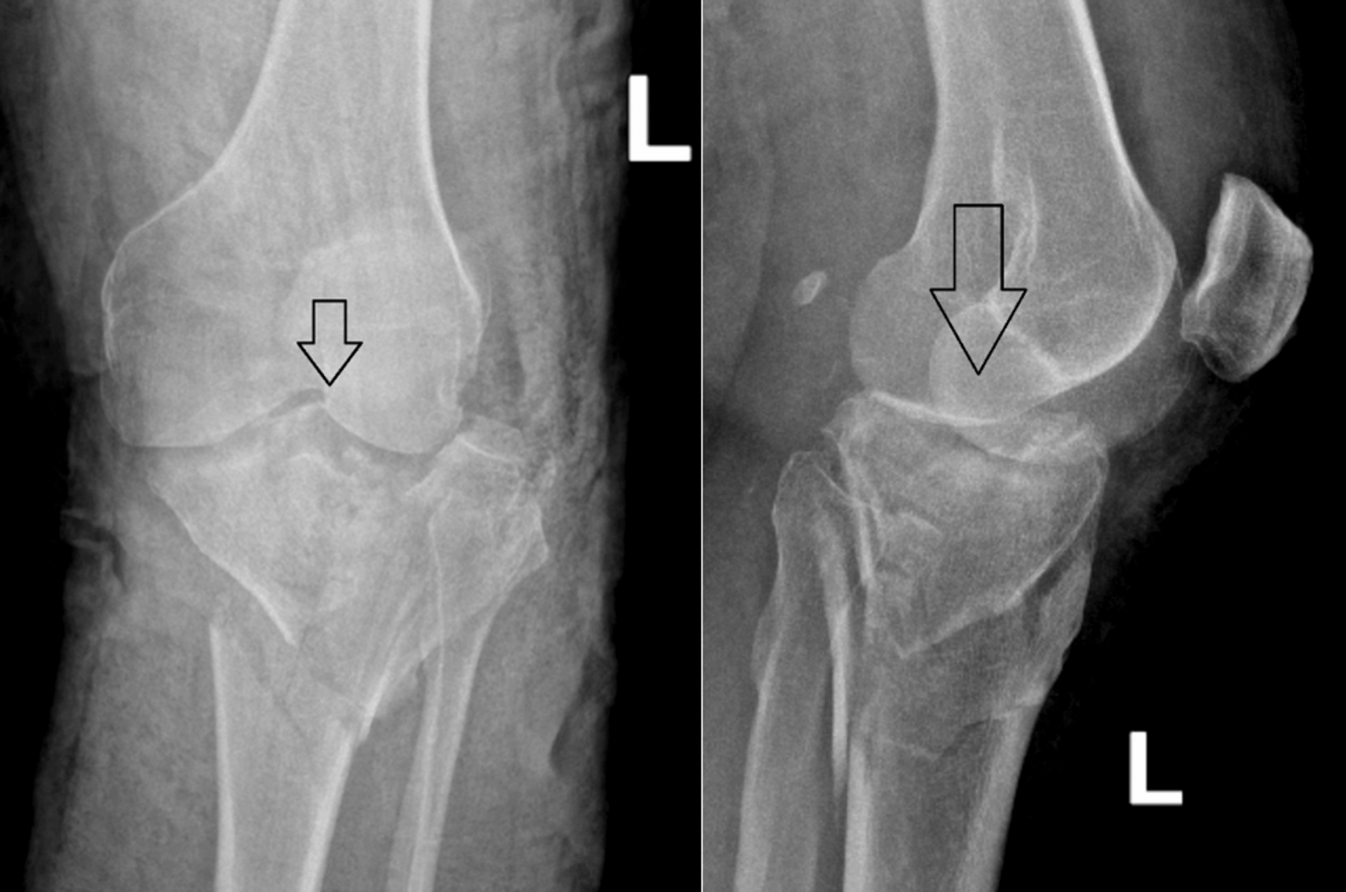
Preoperative X-ray Plateau fracture type V per Schatzker
